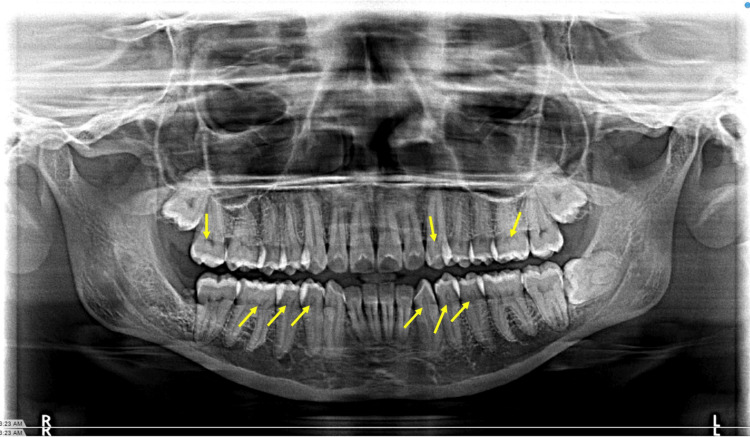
Sample OPG showing pulp stones in maxillary and mandibular teeth. Yellow arrow pointing at the pulp stone. OPG: orthopantomographs
